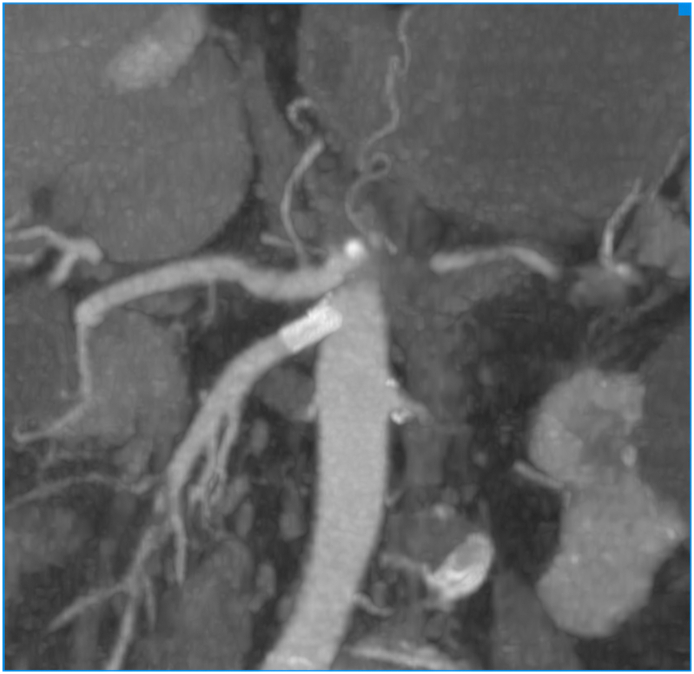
Post-operative CT-angiography showing superior mesenteric artery (SMA) after proximal stunting and mechanical thrombectomy.
